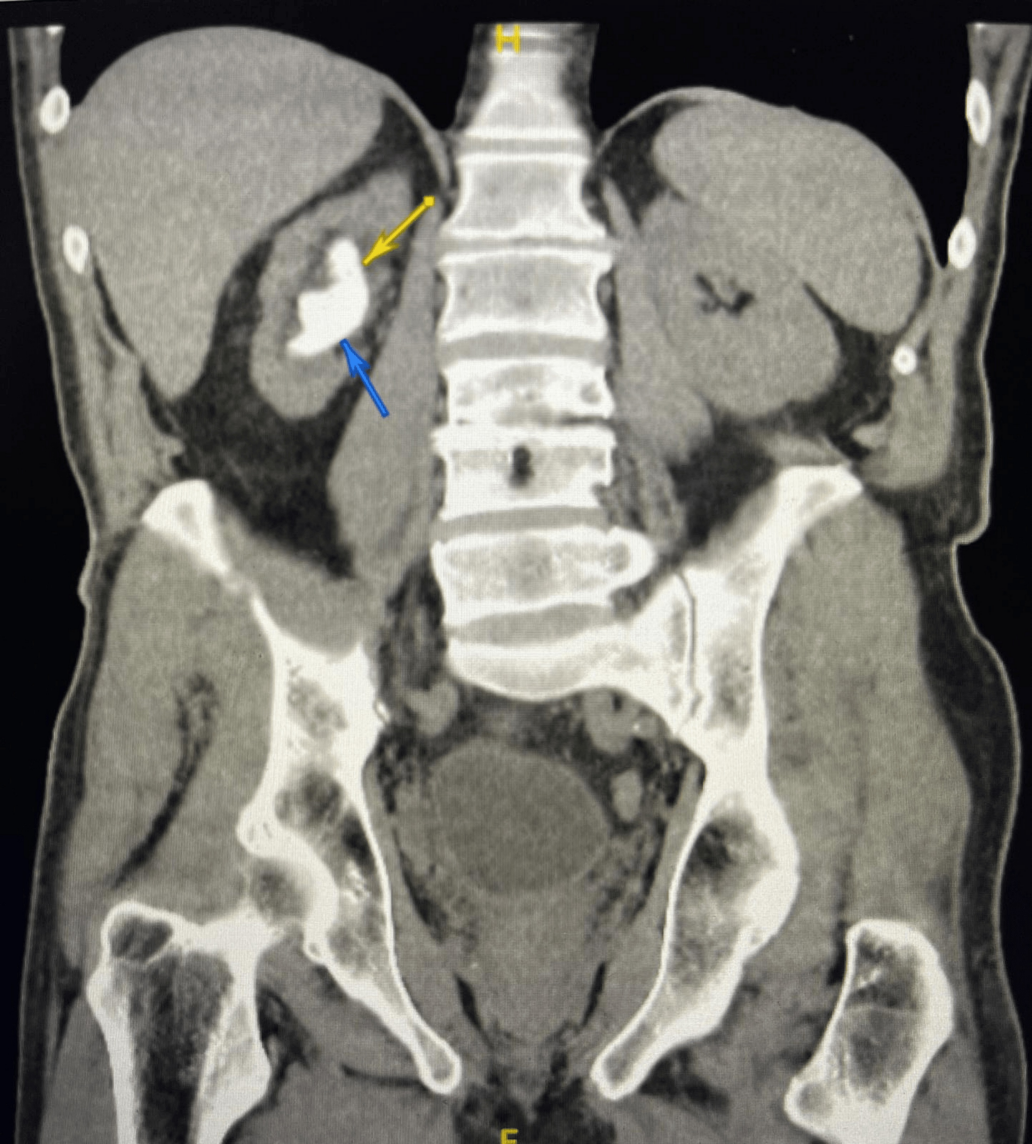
Right staghorn calculus on CT Coronal non-contrast computed tomography scan demonstrating a large right-sided staghorn calculus occupying the renal collecting system (arrows), with associated cortical thinning consistent with renal atrophy.
